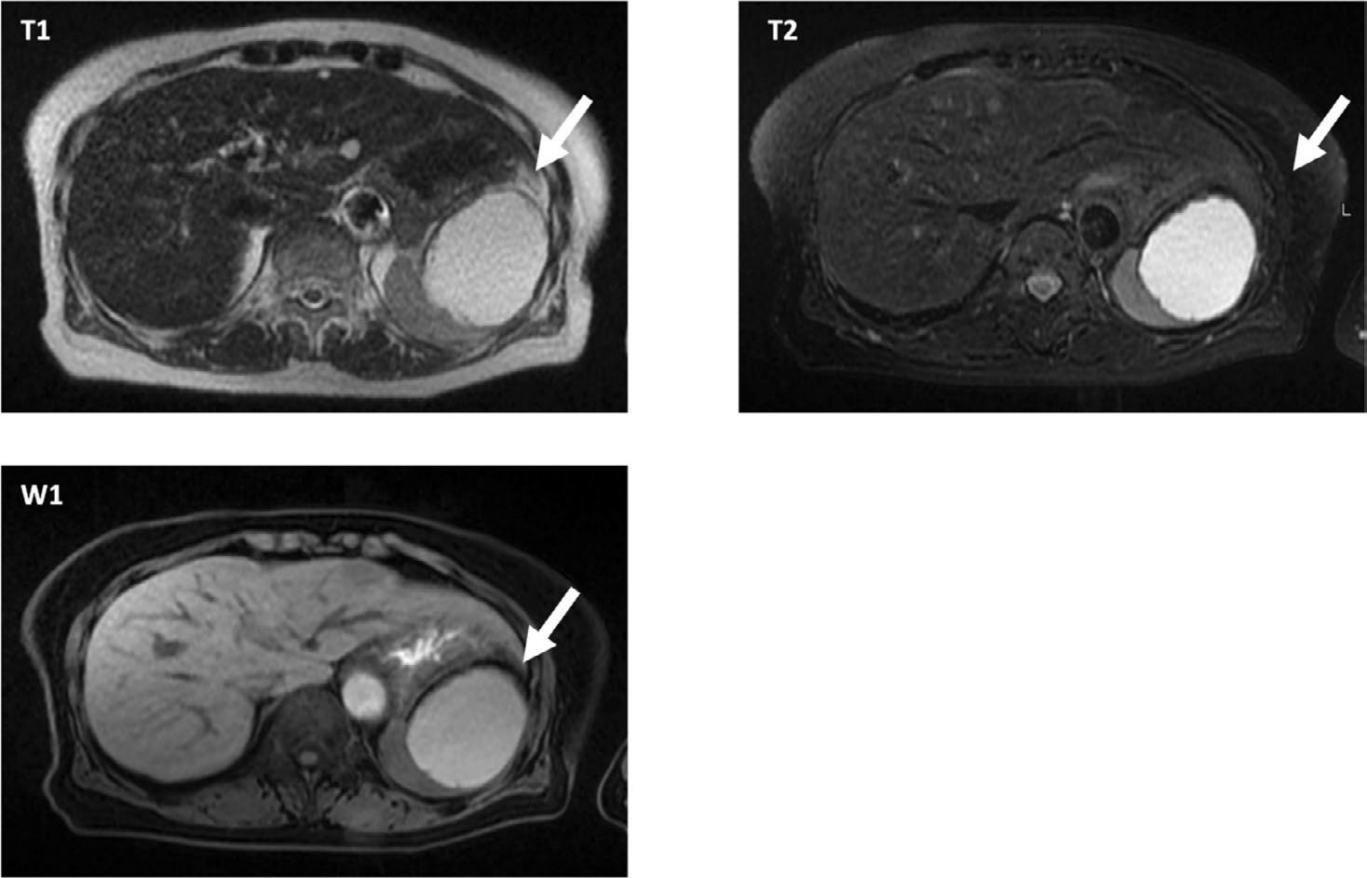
Magnetic resonance imaging with contrast in 2021 showing high FsT1W1 signals in the interior, suggesting the presence of hemorrhagic components.
